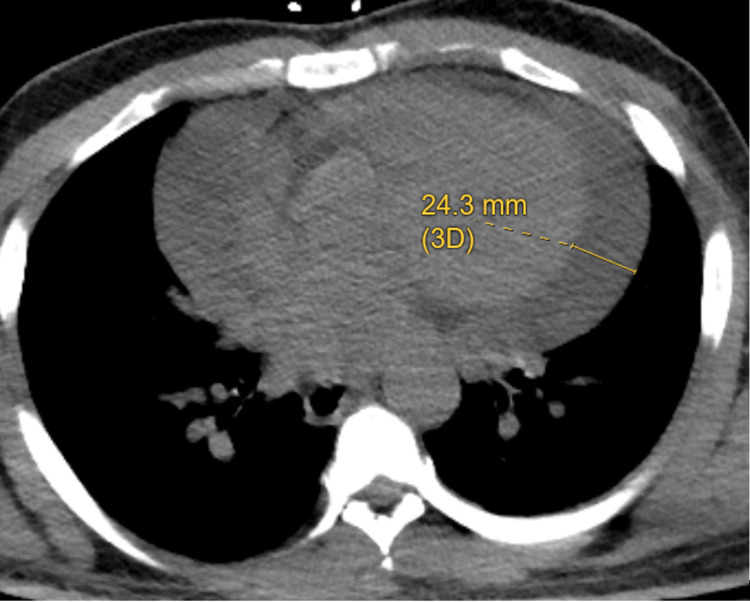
Computed Tomography Chest Pericardial effusion of 24.3 mm (3D) shown on computed tomography chest imaging. D: dimension.
